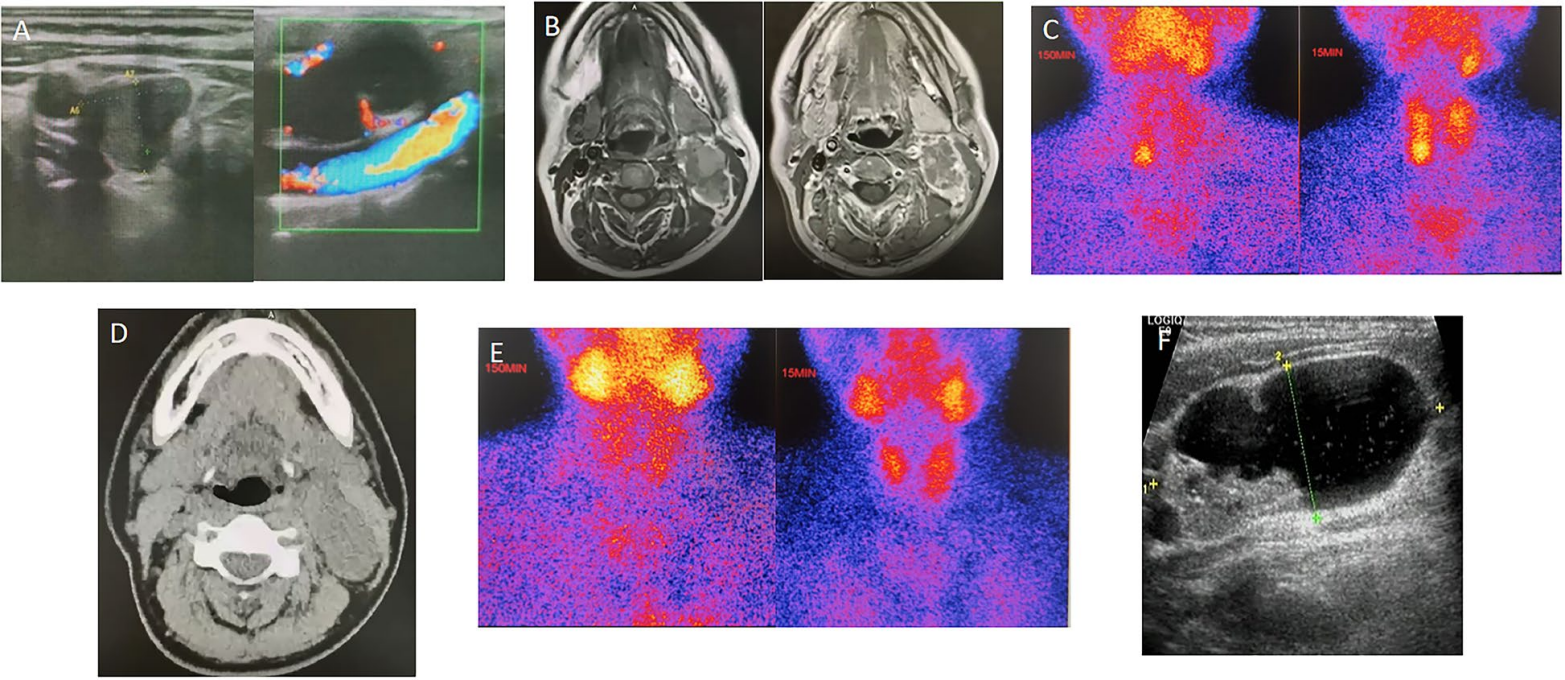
US showed a mass close to the lower side of the right thyroid lobe and a cystic solid mass in the sheath of the left carotid artery (A); MRI showed a cystic solid mass in the sheath of the left carotid artery, about 48 mmX25 mmX39 mm in size, with clear boundary. The solid part showed slightly low signal intensity on T1WI and slightly high signal intensity on T2WI, and the cystic part showed uniform high signal intensity on T1WI and T2WI (B); MIBI showed an uptake in the right inferior parathyroid mass (C); CT revealed a 46 mmx23 mm mass in the sheath of the left carotid artery (D); The postoperative MIBI examination was negative (E); The neck US image of the left neck mass (F)
